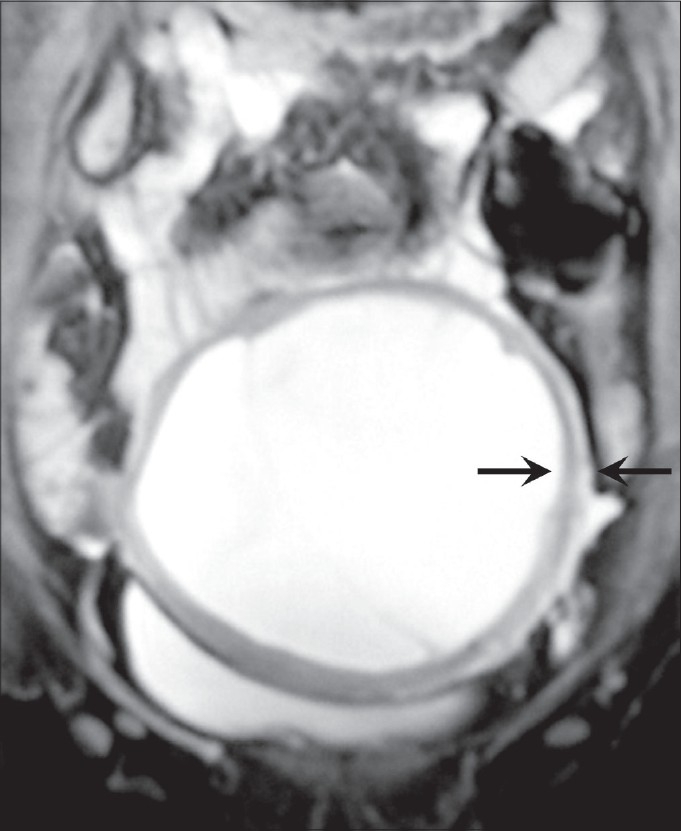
Coronal T2W fat-suppressed MRI shows a hypointense wall (black arrows) and hyperintense internal contents, suggestive of cystic degeneration
